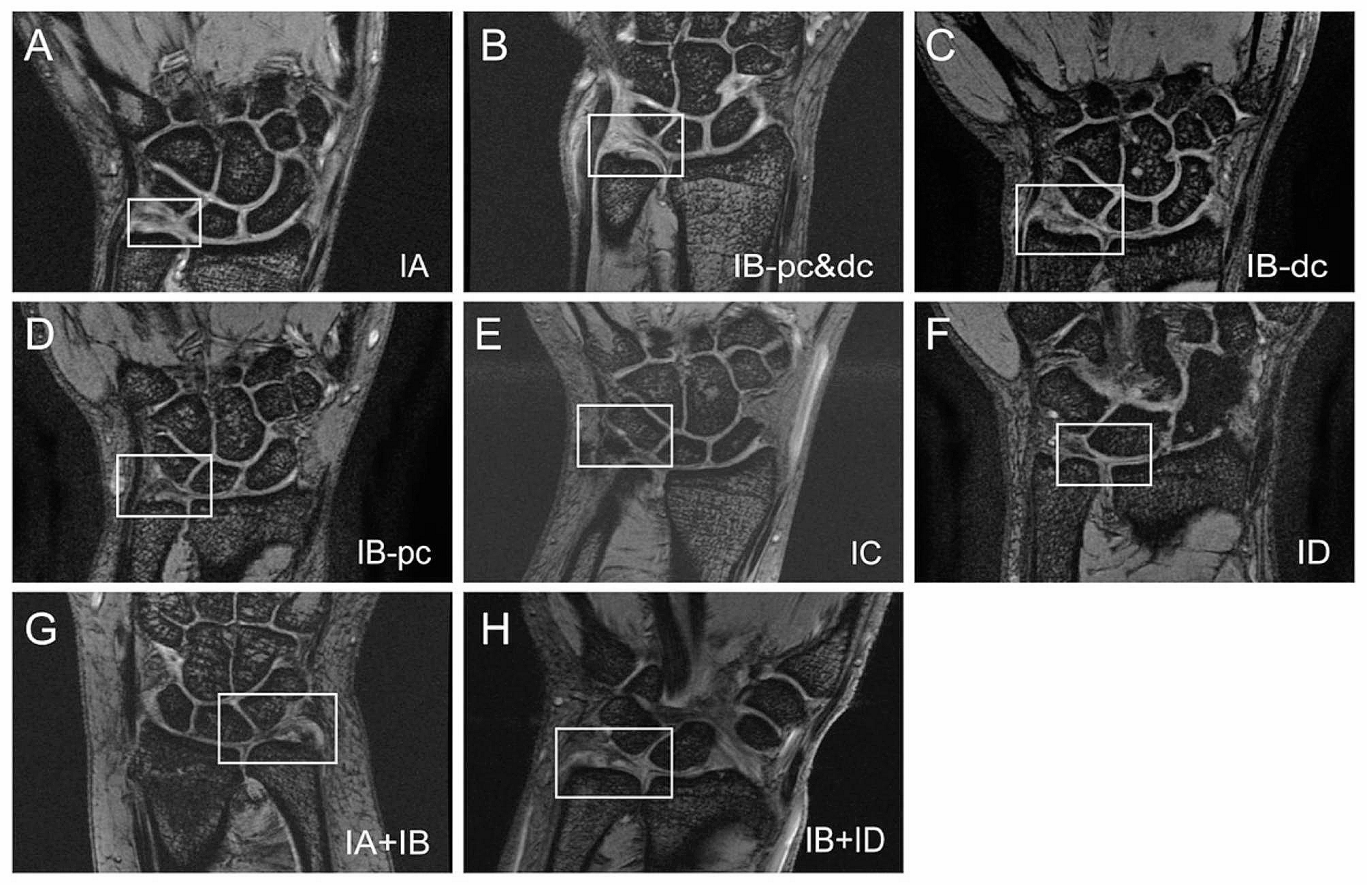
MRI images of the traumatic TFCC injuries according to Palmer’s classification. pc, proximal component; dc, distal component; MRI, magnetic resonance imaging; TFCC, triangular fibrocartilage complex
